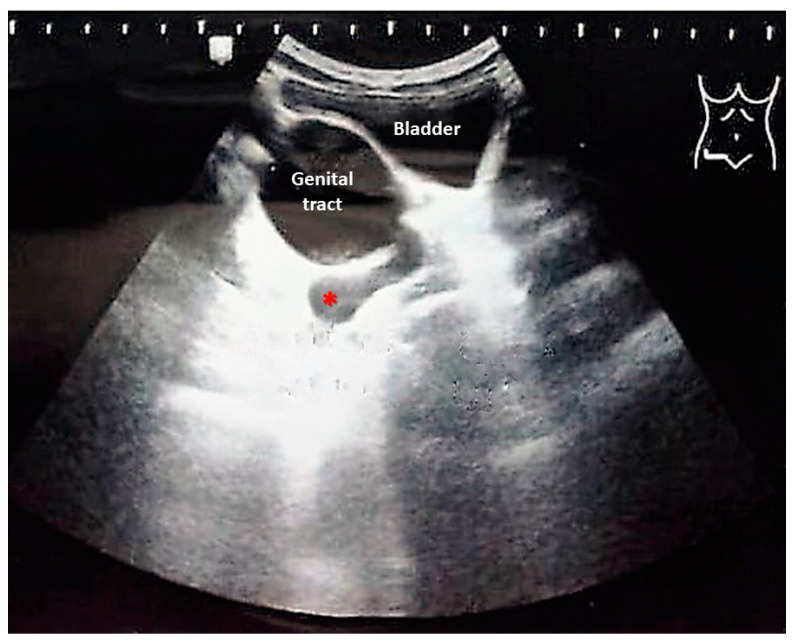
Abdominal ultrasound showing the genital tract filled with fluid, as well as hydrosalpinx (red asterisk).
